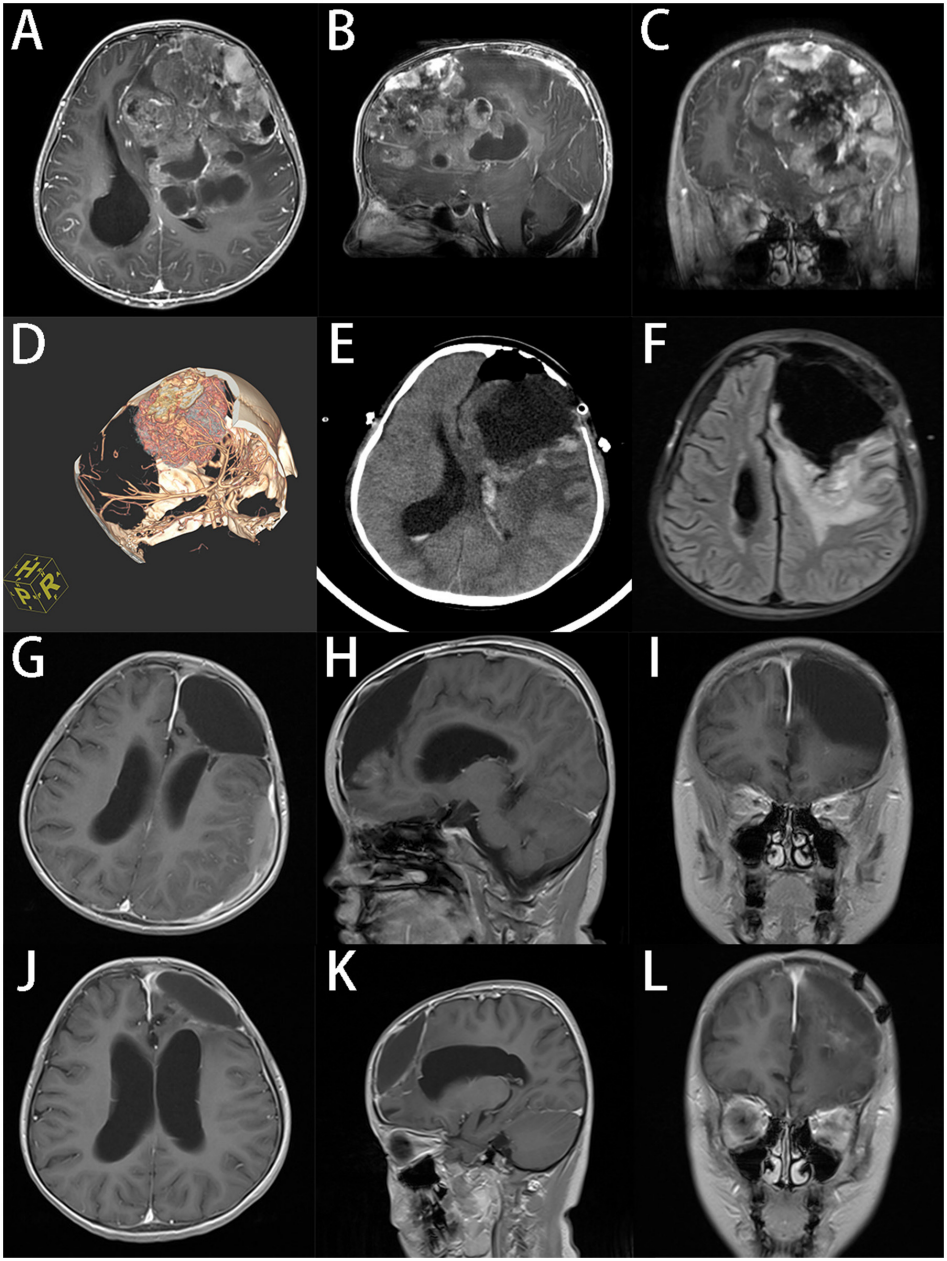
An 8-years-old girl had a giant ependymoma in the left frontal lobe, the tumor volume was 255.8 ml. (A–C) Preoperative axial, sagittal, and coronal gadolinium-enhanced T1-weighted MRI scan shows an inhomogeneous enhanced tumor with hemorrhage necrosis and calcification. (D) 3D reconstruction of CT angiography. (E) Postoperative CT in 24 h after operation. (F) Axial MR at 7d after operation. (G–I) Axial, sagittal and coronal T1-weighted MRI scan indicates that the tumor was totally removed at 3 months after operation. (J–L) Axial, sagittal and coronal T1-weighted MRI scan at 6 months after operation, the residual cavity of tumor reduced.
